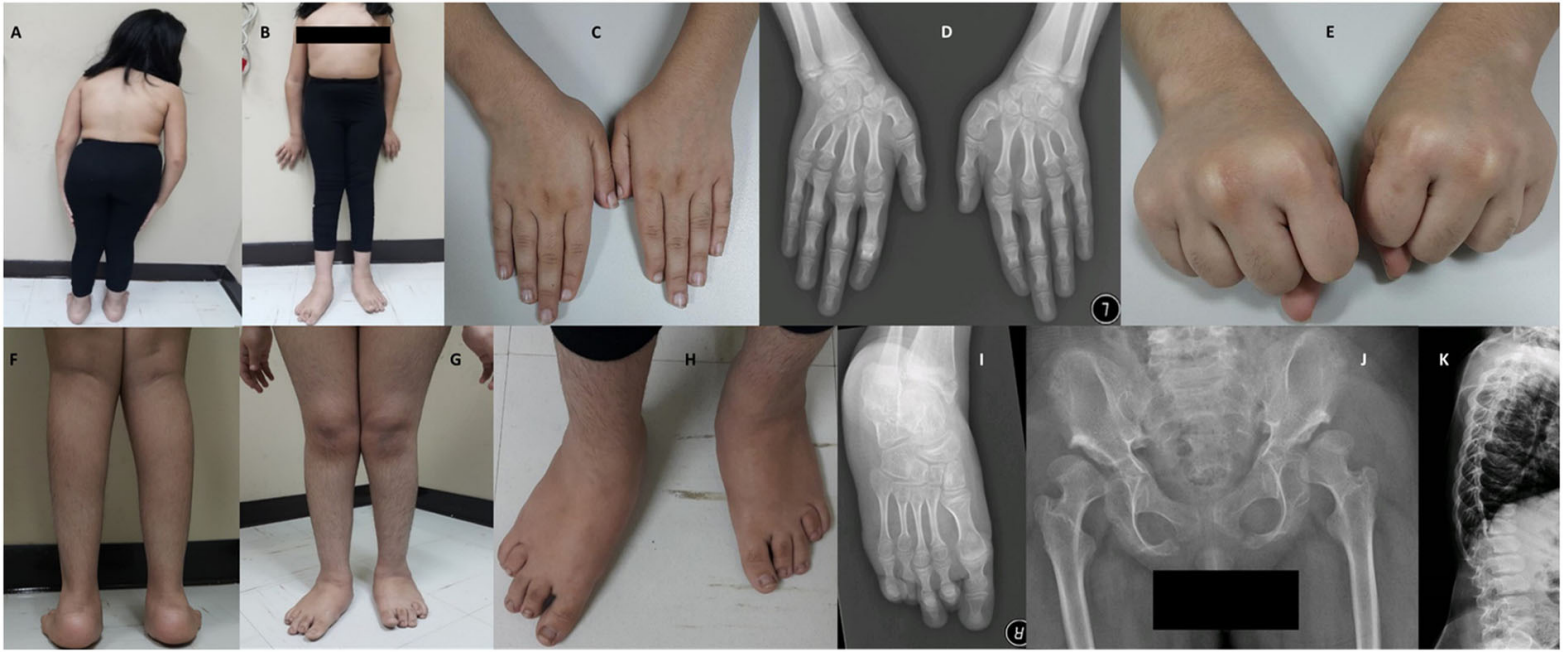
Photos and X-rays of Case-I (IV-7) are showing short trunk, coxavara, mild genu valgum (A,B) short first, fourth and fifth fingers and metacarpals (C,D) and absent fourth and fifth knuckles during fist formation (E), prominent heels, pes planus, brachydactyly of toes more prominent 4th and 5th with short metatarsals (F–I), A-P hips X-rays shows bilateral shallow saucerized acetabulum with abnormally flat and enlarged femoral head and narrow femoral neck, lace-like small iliac wings and bilateral coxa valga (J). X-rays of spines show double hump appearance with antero-inferior beaking of the vertebral bodies (K).
